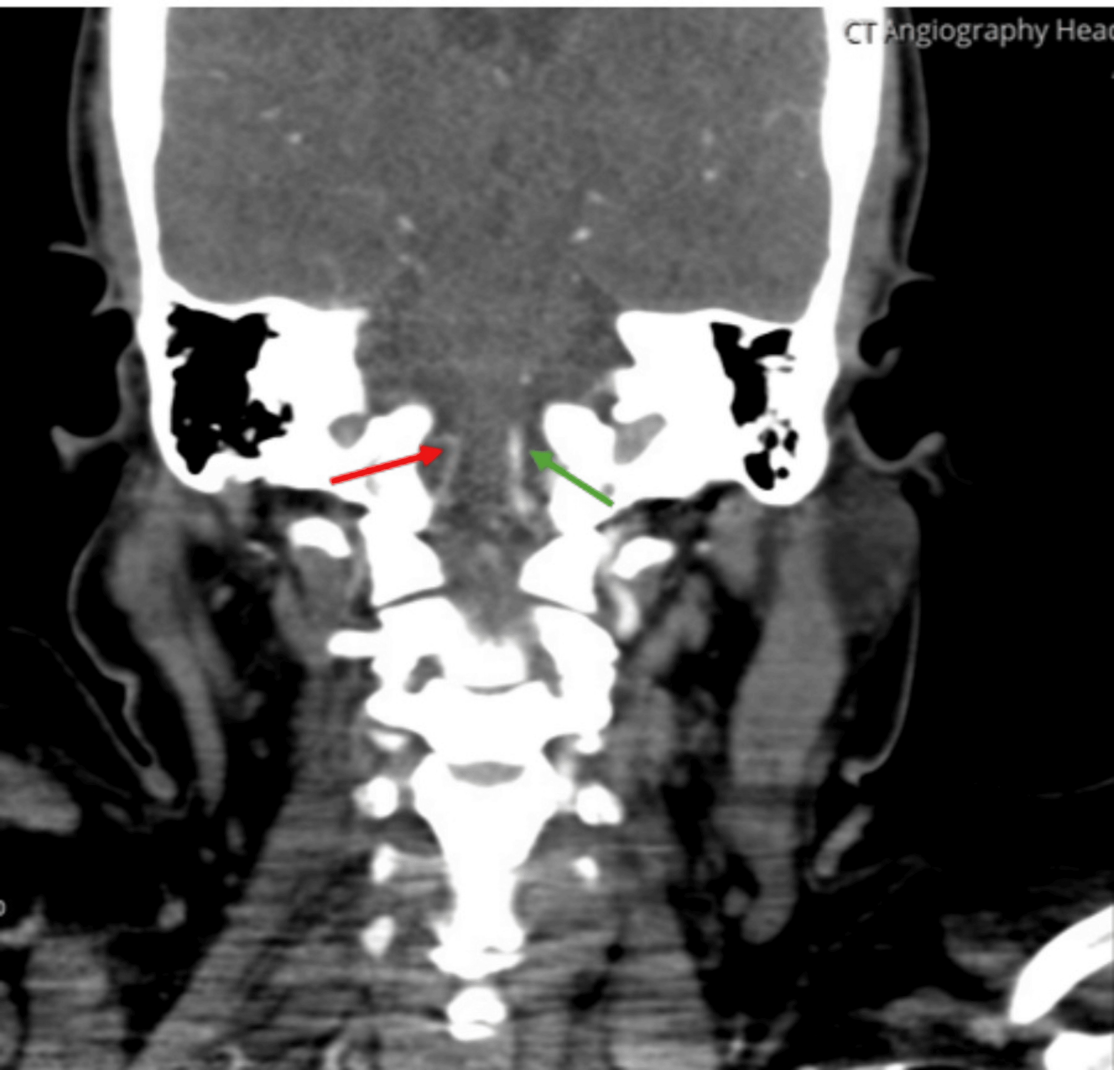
Right vertebral artery dissection on CTA Computed tomography angiography (CTA) of the neck shows reduced enhancement and narrowing of the right vertebral artery (red arrow) compared to the normal left vertebral artery (green arrow), consistent with acute dissection.
